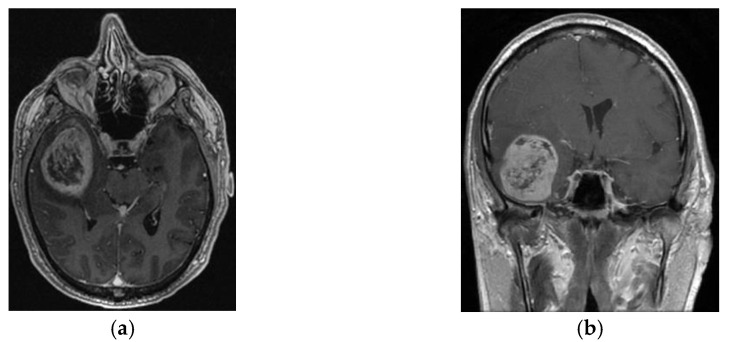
(a) (left) Axial and (b) (right) coronal MRI TI-weighted with contrast preoperative images from September 2019.
